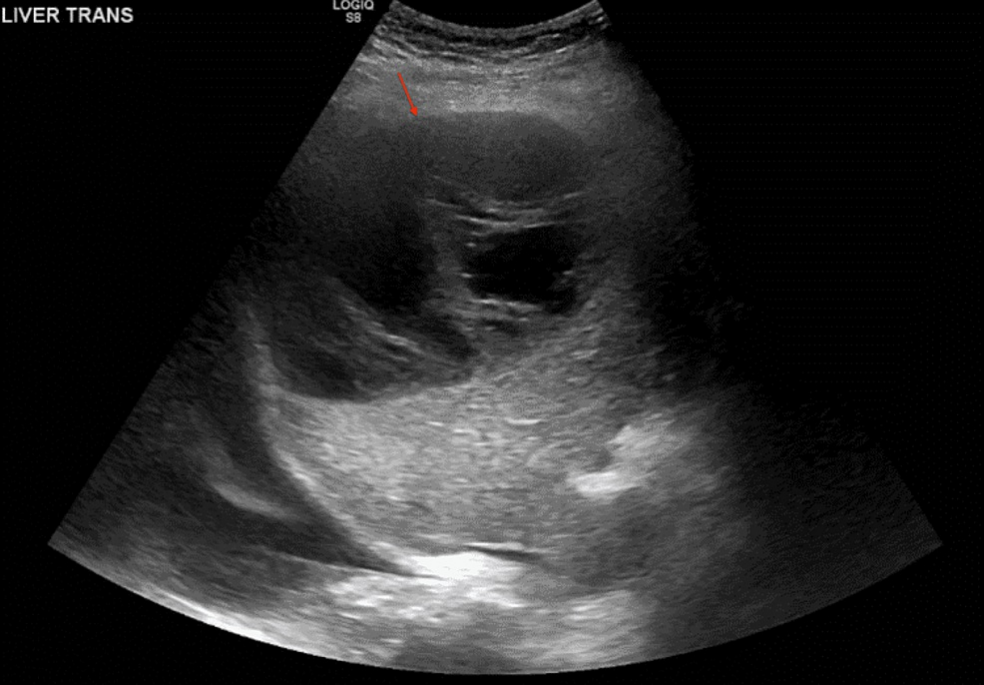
Ultrasound showing well-defined right hepatic lesion with heterogenous echogenicity with thick internal septations.
